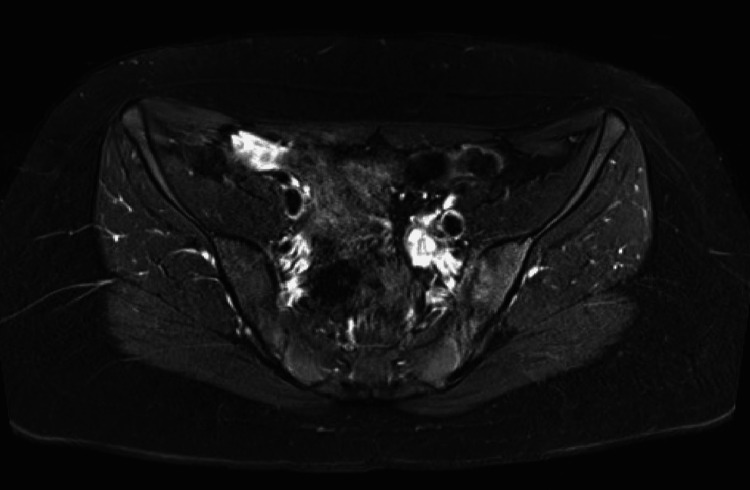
Post-treatment pelvic MRI demonstrating resolution of left sacroiliac infection with mild residual joint enhancement
